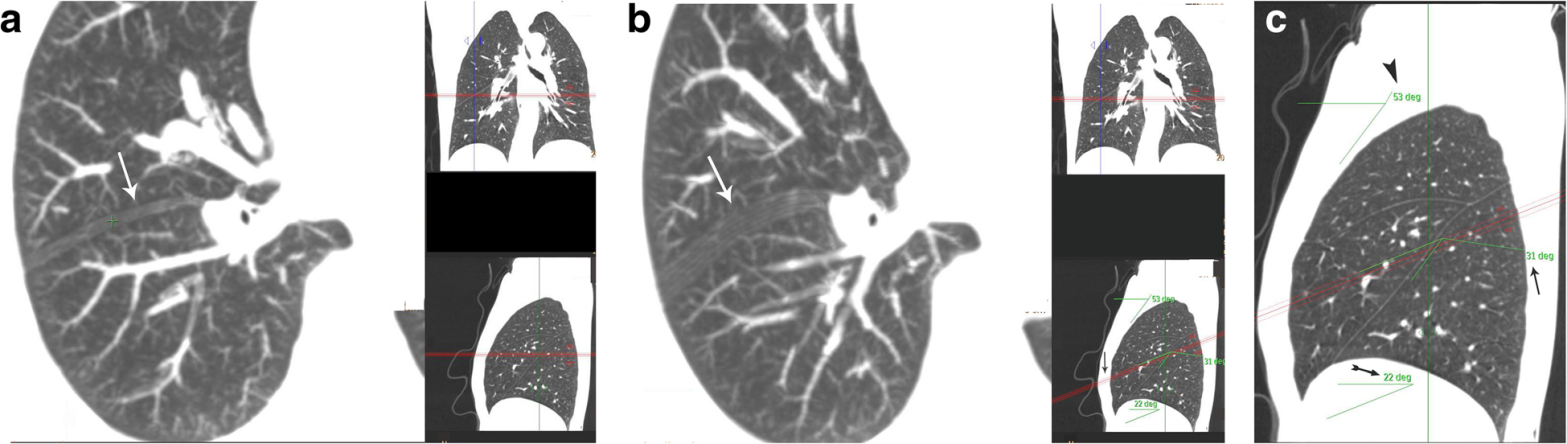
Images from 256-slice spiral computed tomography (CT). a-c Standard algorithm-generated images. Width of the lung window, 1000 Hounsfield units (HU); window level, − 700 HU. a At the workstation three-dimensional interface, the *left* image is an axial maximum intensity projection (MIP) reconstruction with 5-mm slab thickness showing the *lower right* oblique fissure. The interlobular fissure manifested a band-like pattern and had high density and a clear boundary. No five-line sign was revealed (*white arrow*). The basic point (*small green cross*) was set at the middle point of the *lower right* oblique fissure, coronal (*right upper* image), and sagittal plane (*right lower* image) corresponding to the middle point of the axial lower oblique fissures. b In the sagittal position (*right lower* image), which is the same one as shown in a, and the workspace interface, the reconstructed *tangent lines* were rotated counter clockwise to a given angle (*black arrow*) to allow formation of an oblique coronal plane (*left* image). For the interlobular fissure of this oblique plane, a five-line sign was clearly revealed (*white arrow*) with a MIP slab thickness of 6 mm. c On the sagittal position, which is the same one as the *right lower* image shown in b, the angle between the *tangent lines* and the oblique fissure (*arrow*) is 31°; the angle between the *horizontal line* and the oblique fissure (*arrowhead*) is 53°; and the angle between the tangent lines and the *horizontal line* (*arrow* with a fish tail) is 22°. Notice that the minor fissure is imaged as an arc line-like pattern on the sagittal plane
